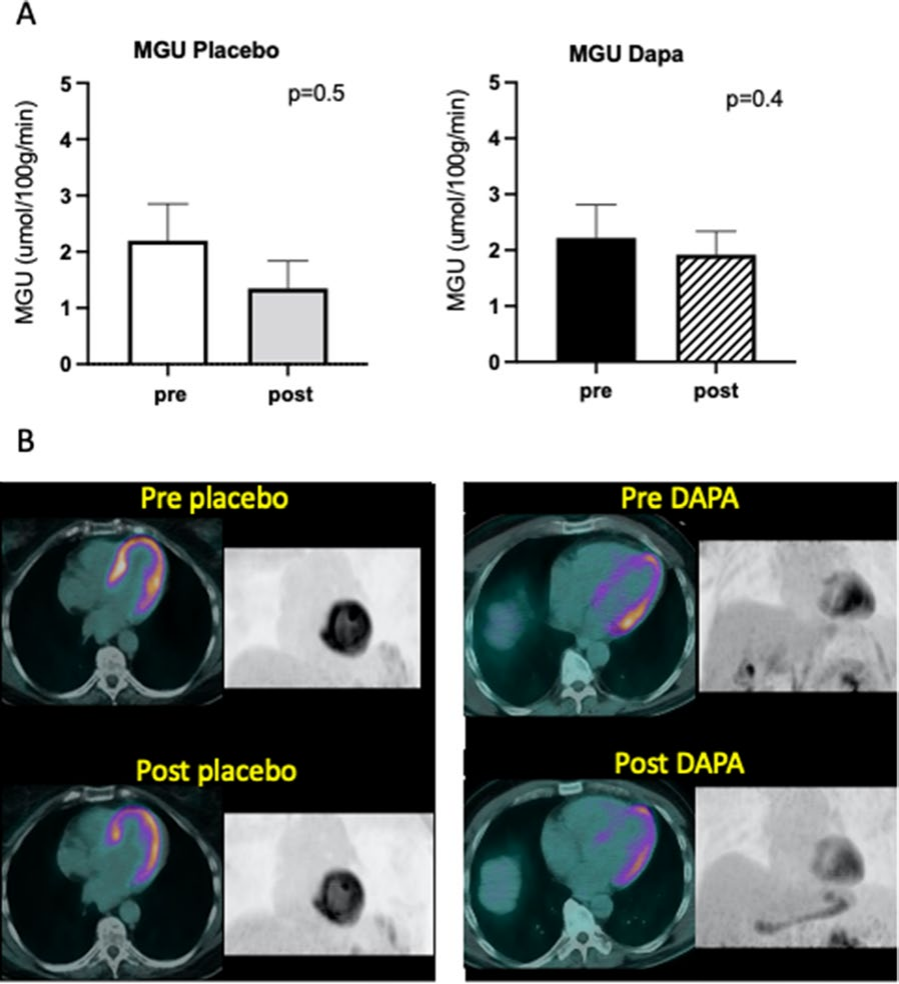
Myocardial glucose uptake (MGU). A Data are mean ± SEM; B FDG PET/CT images during euglycemic hyperinsulinemic clamp of two representative cases: fused and 3D maximum intensity projection anterior views pre- and post-placebo and pre- and post-dapagliflozin, respectively
